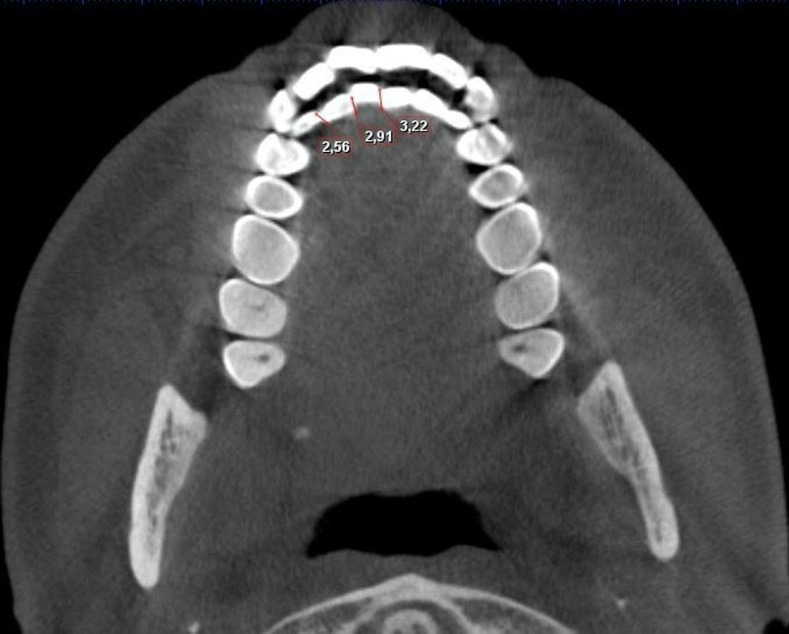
CBCT axial view showing the measurement of LII.
